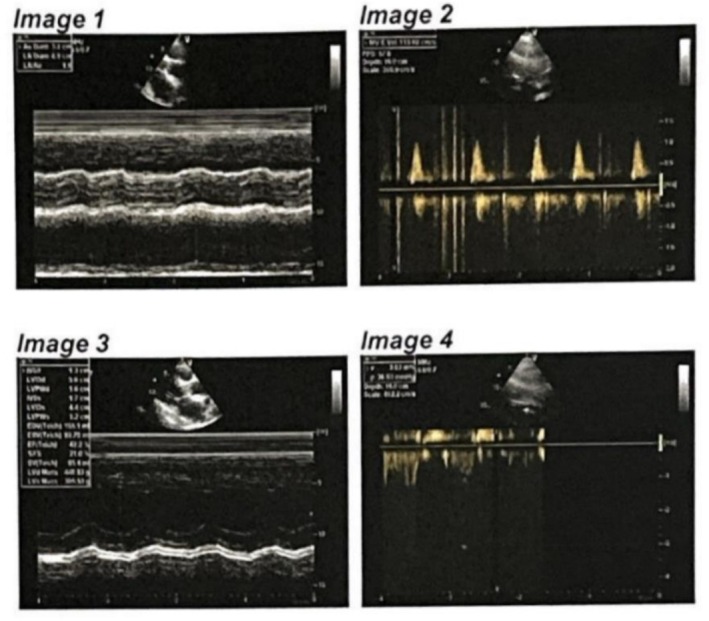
Echocardiogram performed upon hospitalization shows a moderately dilated left atrium (4.9 cm), moderately impaired left ventricular systolic function (EF = 42.4%), and an elevated right ventricular systolic pressure (40–45 mmHg).
